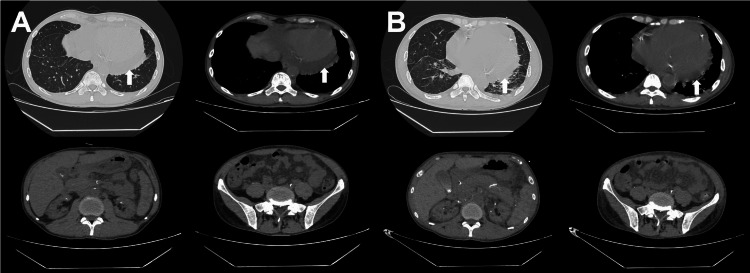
CT Scans of the Chest and Abdomen (A) CT obtained on February 6, 2023. (B) CT obtained on February 10, 2023. Lungs: Increased bilateral pulmonary edema and pneumonia. Pleural effusion on both sides. Heart: Left ventricular enlargement is now evident. Pericardial effusion remains stable. Abdomen/pelvis: Development of peritoneal and mesenteric edema. Small ascites (pelvic and peritoneal). Spleen: Multifocal hypodense lesions, compatible with splenic infarcts. CT: computed tomography
